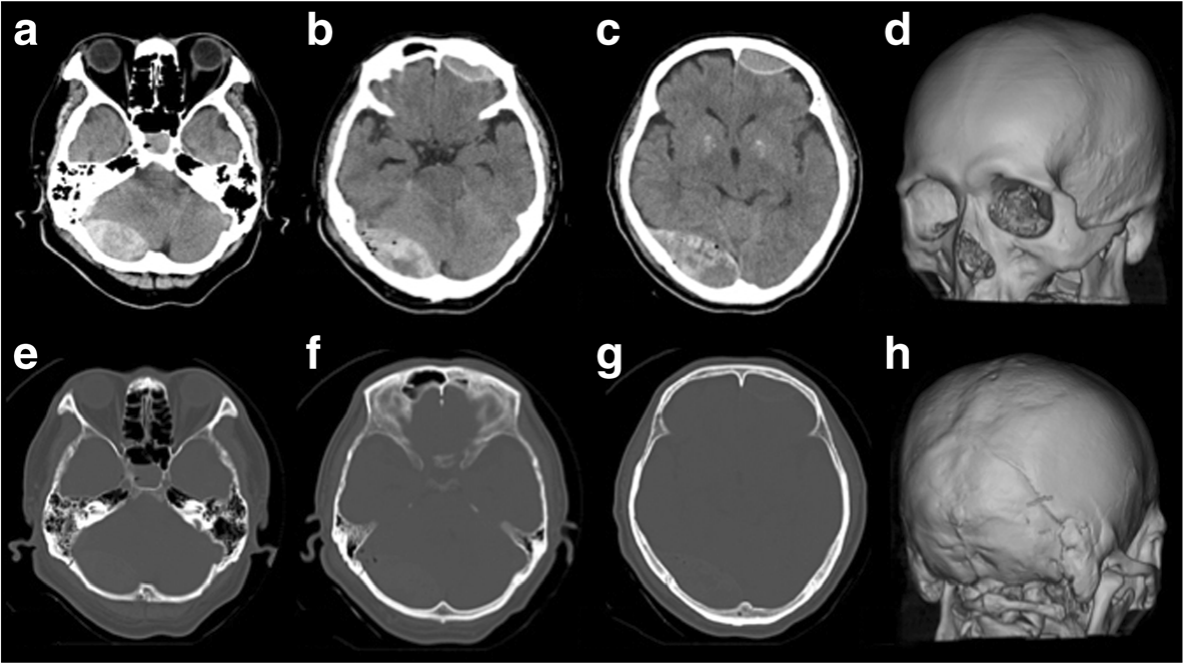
Head computed tomography (case 2) on admission. Computed tomography showed acute epidural hematoma in the right occipital, right suboccipital, and left frontal region (a-c). There was a separated skull fracture on the lambdoid suture (d-h)
